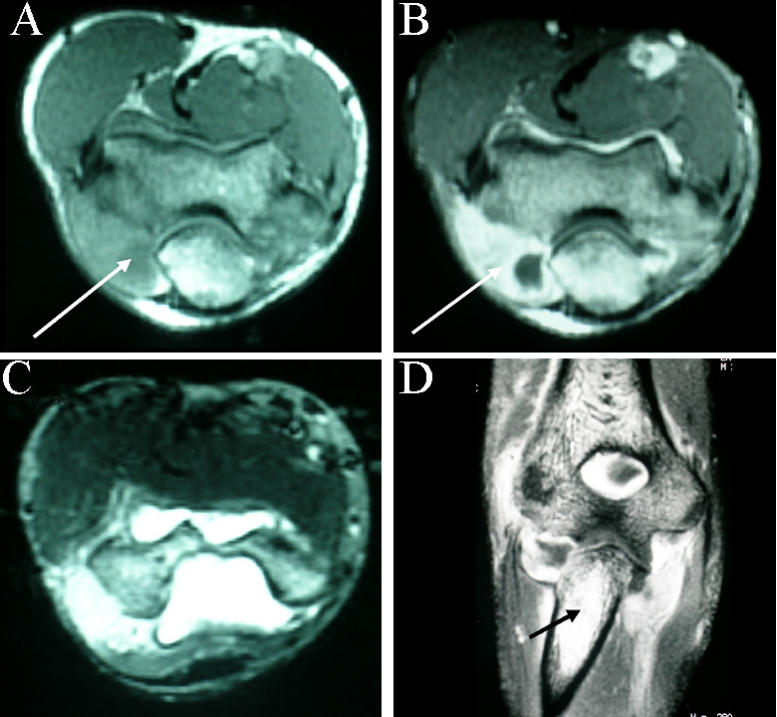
Multiplanar CT of the right elbow joint. A. Axial view showing a complex joint effusion (arrowed) and soft tissue swelling adjacent to the elbow. B. Axial slice through the proximal forearm, demonstrating the joint effusion surrounding the radial head (solid arrow) and increased soft tissue density involving the medullary shaft of the ulna (dashed arrow). C. Sagittal bone targeted reconstruction, showing subtle cortical erosions (arrowed) of the proximal ulna. D. Sagittal soft tissue reconstruction, demonstrating increased soft tissue density (arrowed) of the medullary shaft of the proximal ulna.
